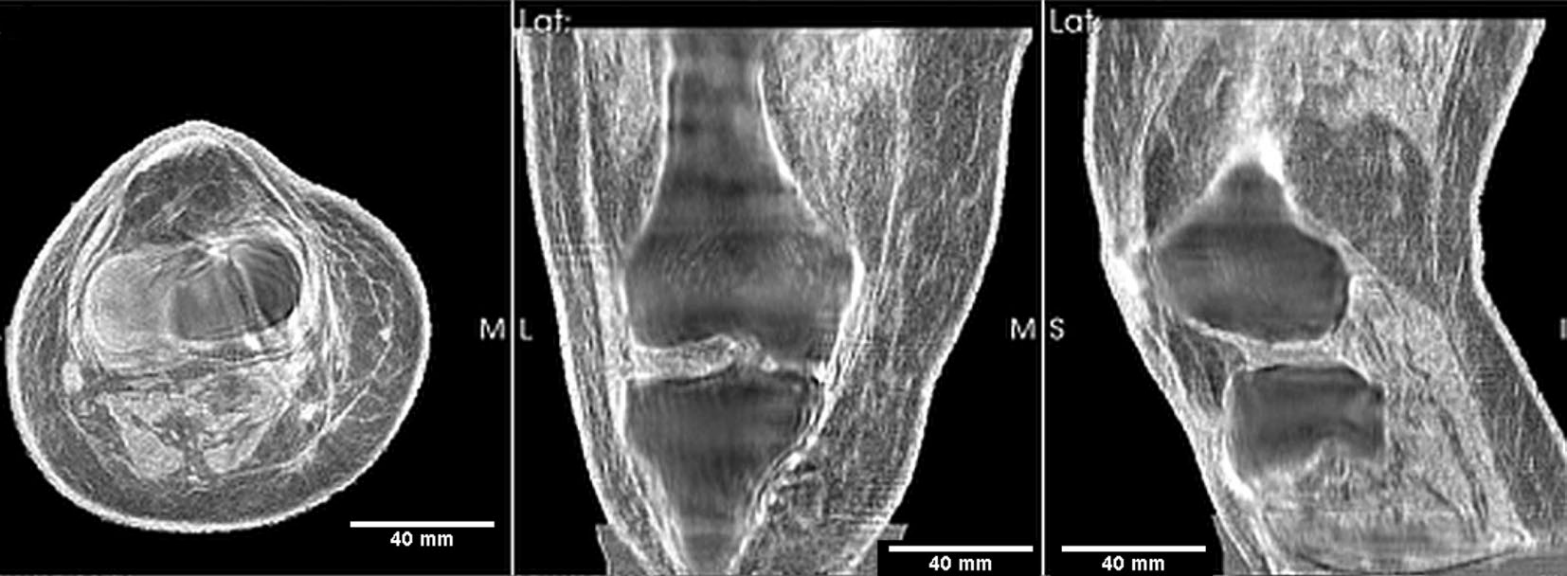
Fused image: axial, coronal and sagittal views of the transmission ultrasound 3D speed of sound map fused with reflection image of mature human knee. This representation highlights the 3D nature of the reconstruction. The articular cartilage in the tibiofemoral space is clearly visible. The lateral and medial collateral ligaments are visible as (correct) high speed regions. The high speed muscle is also shown with the correct SOS being read off of the perfectly registered pure SOS (speed of sound) image.
